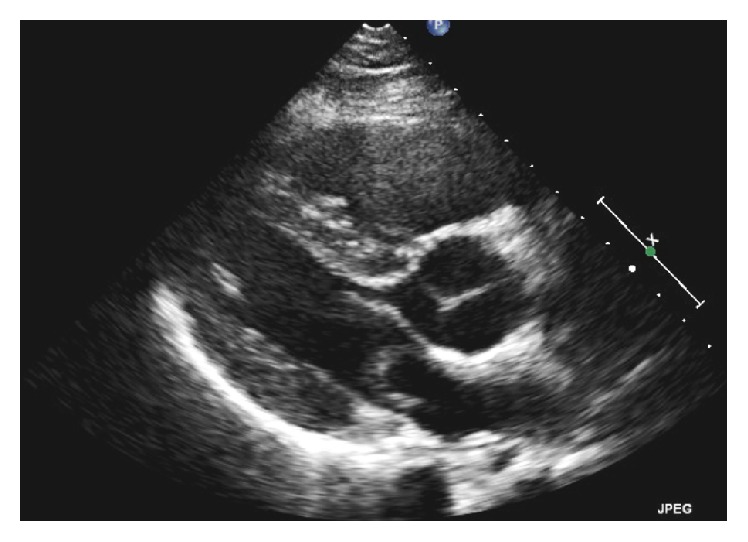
Echocardiogram showing mild concentric left ventricular hypertrophy and a serpentine mass in the left atrium, consistent with thrombus.
